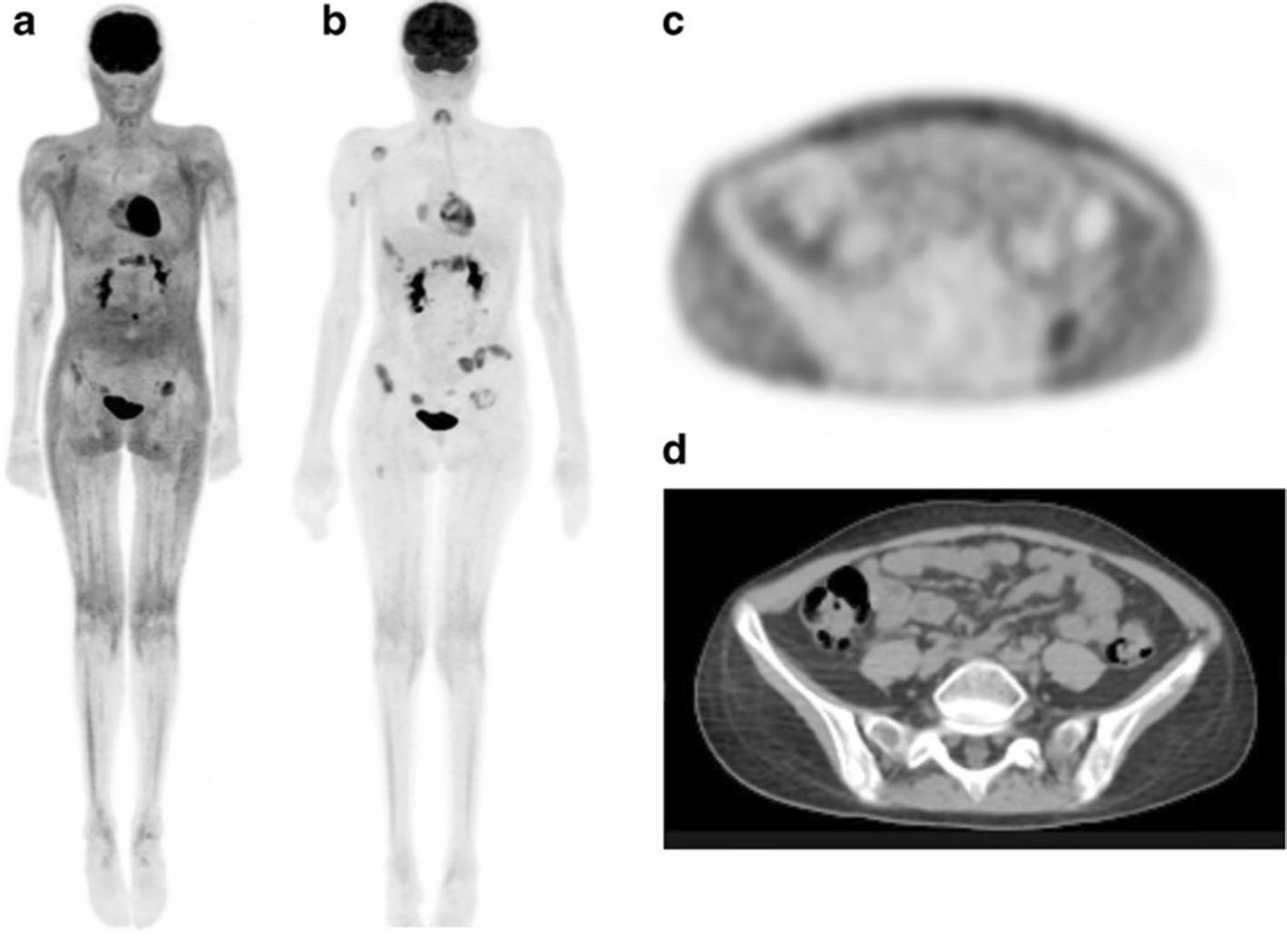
FDG-PET/CT maximum-intensity projection (MIP) in a 14-year-old patient as presented by Wong et al. with acute myeloid leukemia showing abnormal biodistribution of FDG localizing to subcutaneous white adipose tissue (A). SUVmax in the white fat of the flanks and gluteal regions was found to be 1.8 (C and D). Repeat FDG-PET/CT one week later (B) showed normalization of FDG biodistribution, revealing metabolically active osseous lesions in the proximal right humerus, right scapula, ribs and right femur, which were previously obscured. Image reprinted without changes from Wong et al. (2020)
